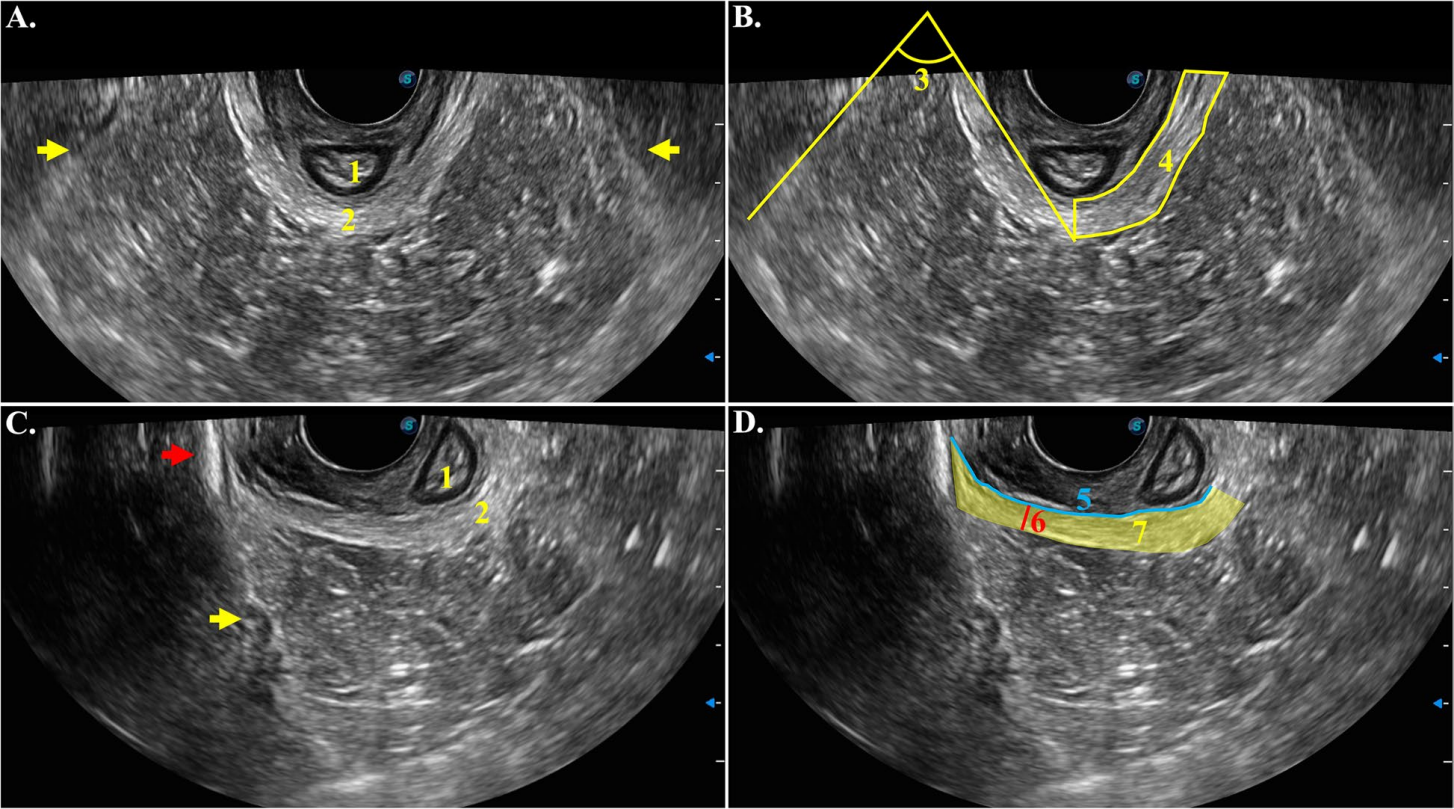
Transvaginal ultrasound showing the axial plane of the puborectalis (PR) in a normal woman. Yellow arrows (A, C) indicate the arch tendinous levator ani (ATLA). The red arrow (C) indicates the pubis branch. 1, anal canal; 2, PR; 3, angle between the ATLA and PR (AAP), 74.386°; 4, measurement region of grayscale of the left PR, indicted by yellow frame. The grayscale is 142.152; 5, length of the right PR, 5.033 cm; 6, minimum width of the right PR, 0.416 cm; 7, area of the right PR, indicated by yellow shade, 2.994 cm^2^
